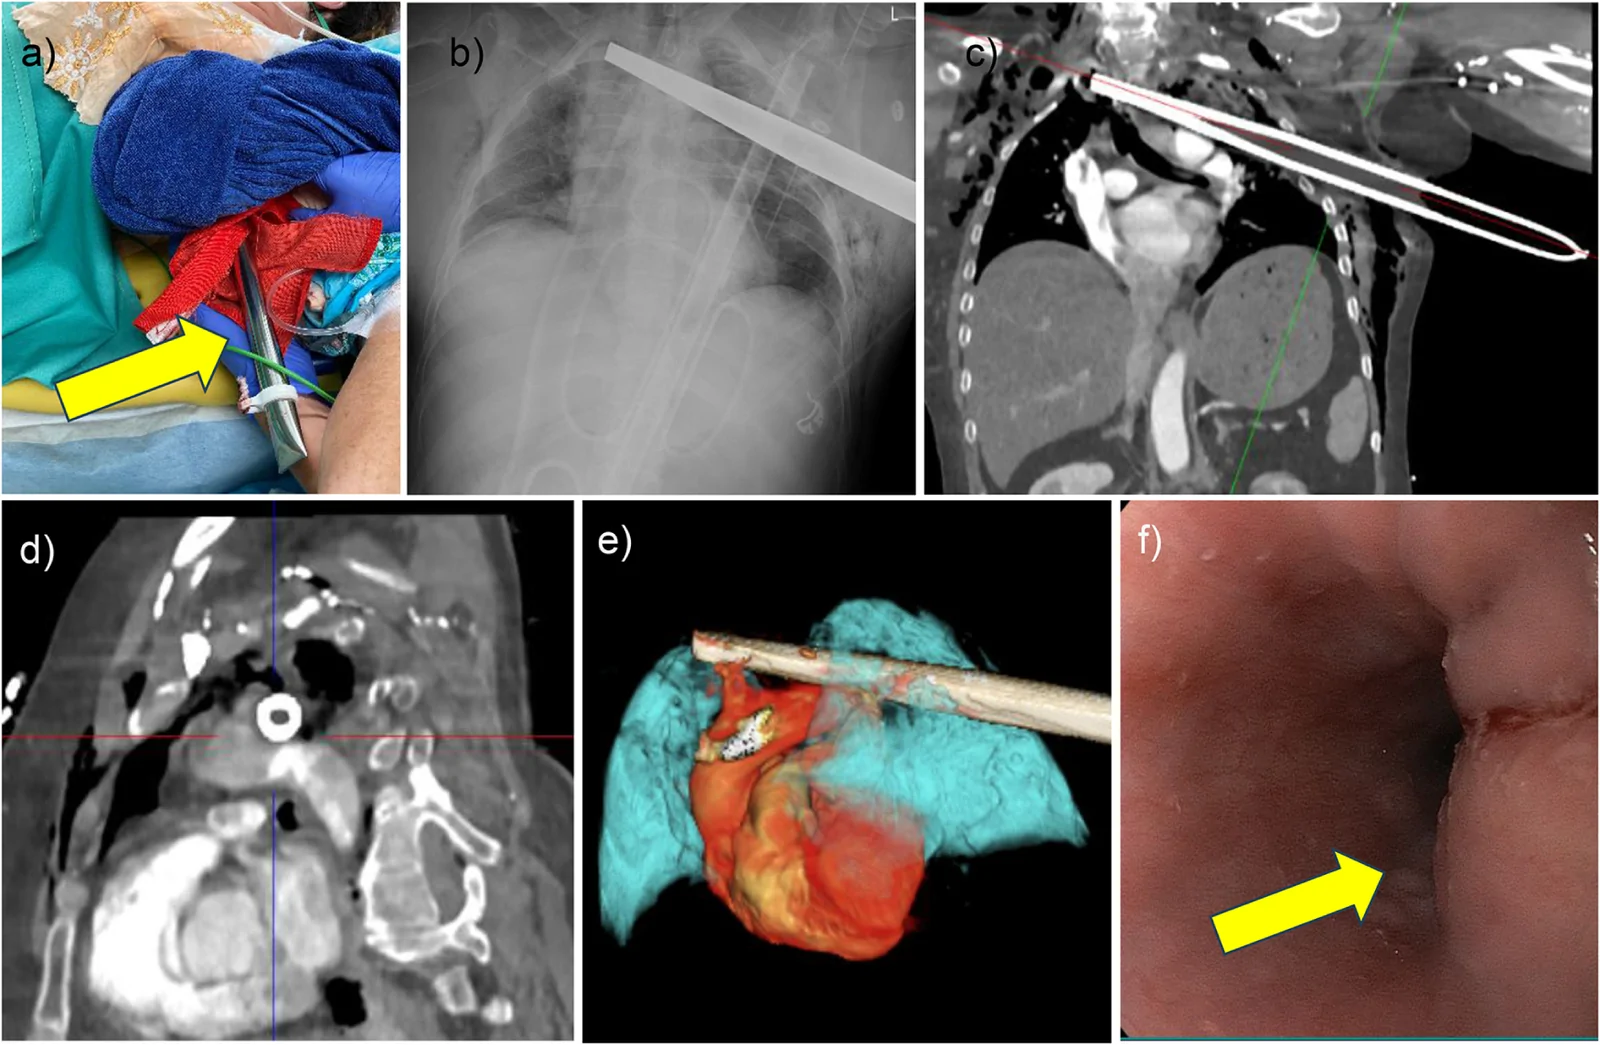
(A) Penetrating flagpole (yellow arrow) manually stabilized throughout trauma management; (B) Initial chest x-ray; (C) Coronal CT scan; (d) Sagittal CT scan; (e) Three-dimensional CT reconstruction demonstrating the position of the flagpole in relation to the lungs and aortic arch; (f) Preoperative esophagoscopy showing extrinsic compression of the ventral esophageal wall (yellow arrow) without evidence of an endoluminal lesion.
